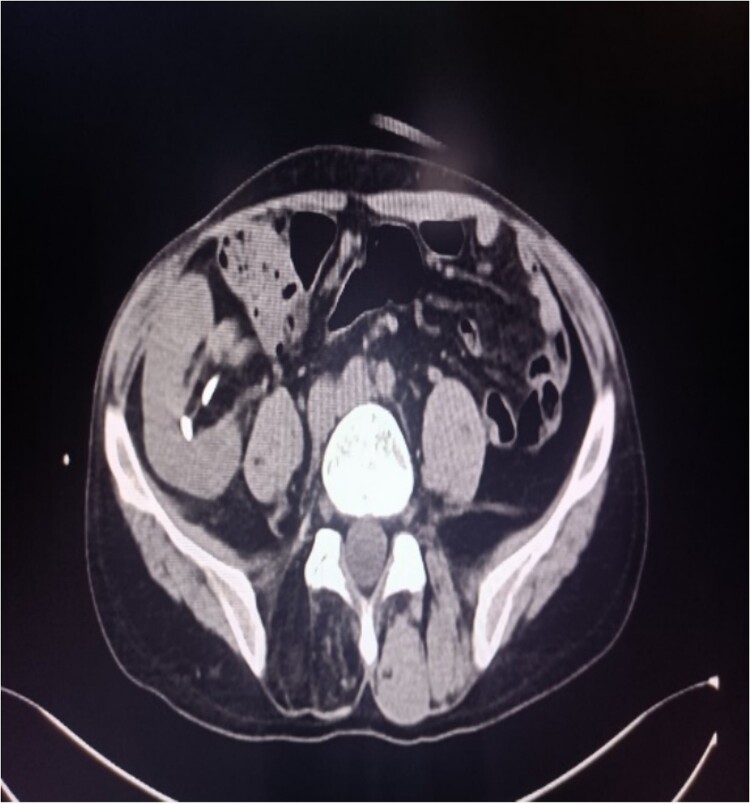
CT of the transplanted kidney shows stones.
